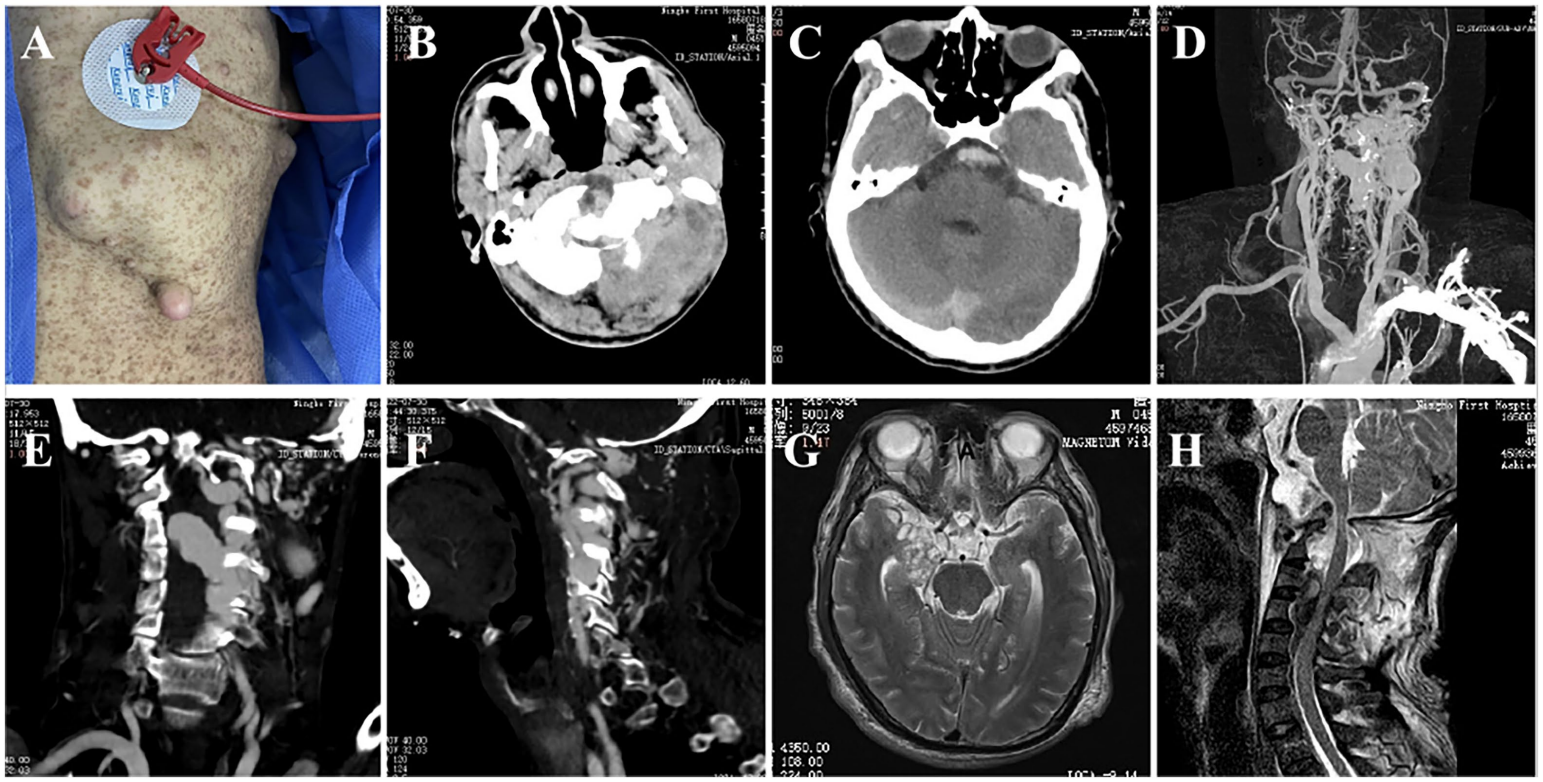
The patient’s body surface features and imaging findings. (A) Multiple neurofibromas and typical café-au-lait spots; (B) Left skull bone destruction and occipital muscle swelling; (C) CTA showed subarachnoid hemorrhage; (D–F) CTA showed multiple vascular abnormalities in the left skull base and neck, and the thick venous lake compressed the spinal cord; (G) MRI showed the presence of an intracranial tumor; (H) Atlantoaxial dislocation, fluid void signal in the spinal canal and compression of the spinal cord.
